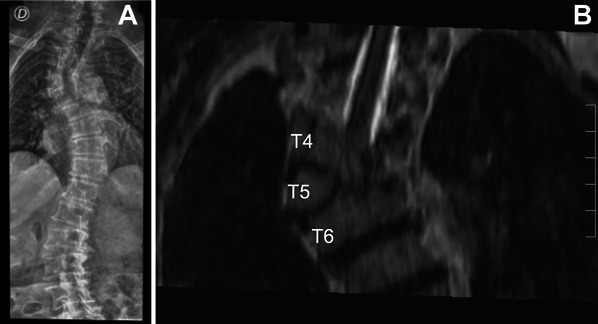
A, Antero-posterior thoracic spine x-rays and B, coronal T2-weighted MRI showing proximal thoracic right convex scoliosis and right T5 hemivertebra.
